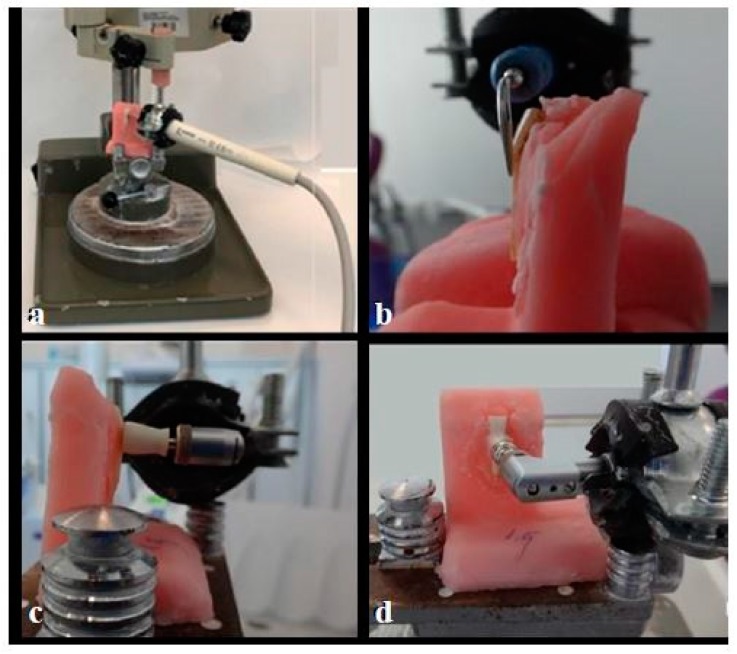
Handpieces fixed to the setup (a) Piezoelectric ultrasonic scaler, (b) Magnetostrictive ultrasonic scaler, (c) Rubber prophy cup, (d) Nylon bristle brush.
